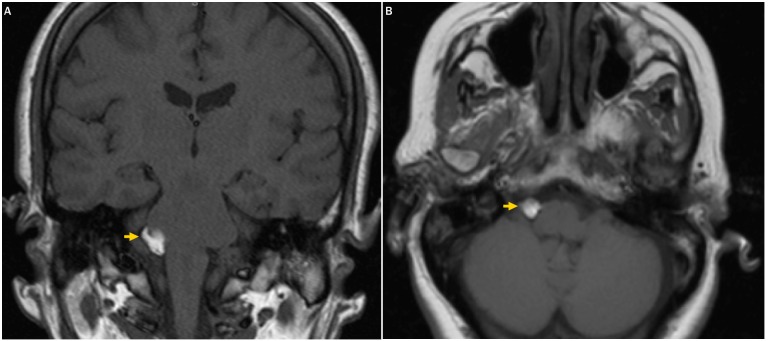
A T1-weighted MRI indicating a right-sided CPA lipoma (A) A coronal T1-weighted MRI and (B) an axial T1-weighted MRI demonstrating a hyperintense mass in the right CPA (yellow arrows) consistent with a lipomatous tumor.
